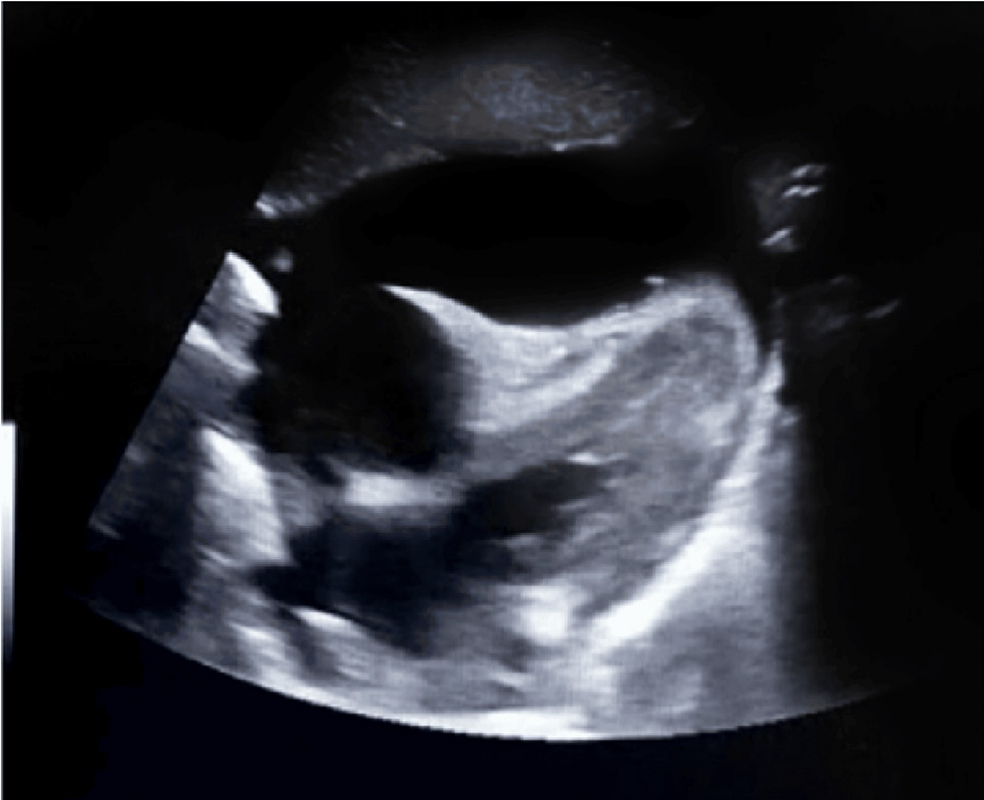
Point-of-care ultrasound: bedside echocardiography subcostal view with right ventricular collapse, which reflected significant pericardial effusion
